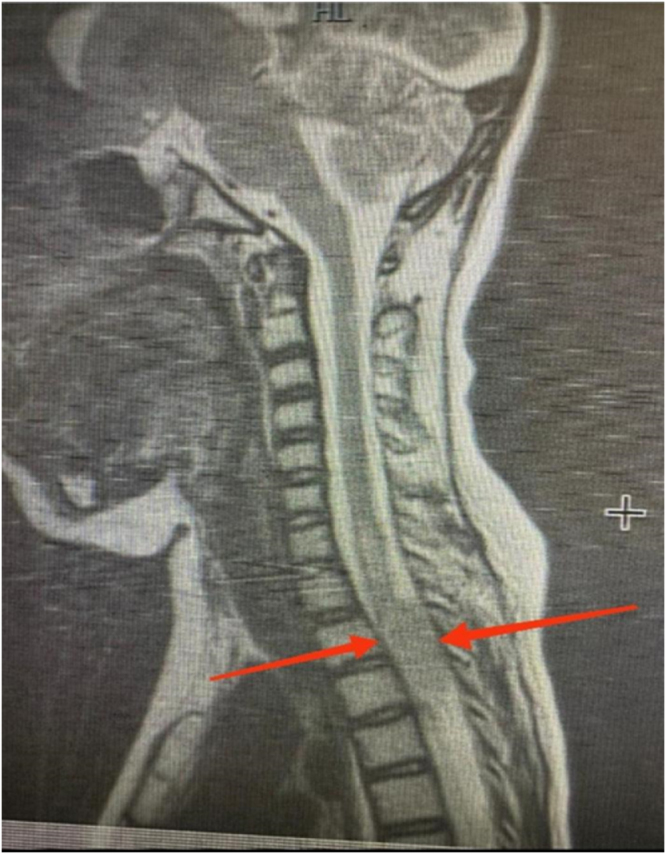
Sagittal T2-weighted MRI of the cervicothoracic spine showing a posterior epidural mass at C7–T1 compressing the spinal cord, consistent with drop metastasis.
